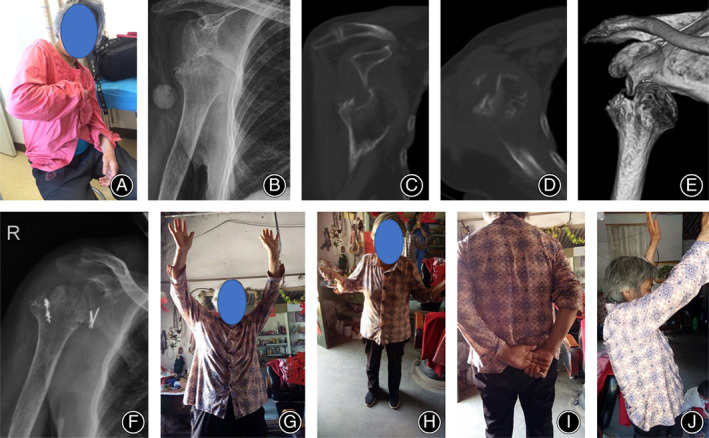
A 69 years old woman with right chronic anterior shoulder dislocation more than 2 months (No.4 patient). (A): This female patient complained severe pain and limited activity. (B): Preoperative anteroposterior radiograph showing a chronic anterior dislocation. (C‐E): Preoperative CT scans showing chronic anterior dislocation with large Hill‐Sachs lesion. (F): Anteroposterior radiograph 3 months postoperatively showing right shoulder reduction with Latarjet procedure and remplissage technique. (G‐J): The right shoulder function of this patient basically returned to normal 2 years after surgery.
